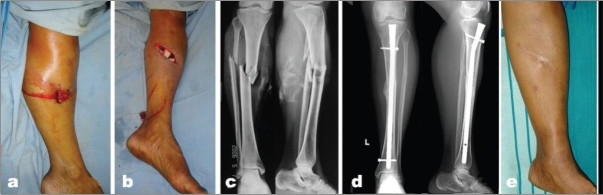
(a & b) Clinical photograph showing open injury of the tibia with exposed bone. (c) X-ray anteroposterior and lateral views of leg bones showing comminuted fracture of tibia. As per the Ganga Hospital Score, the total score is less than 5 and the skin score is less than 3. (d) X-ray anteroposterior and lateral view of leg bones showing skeletal fixation and union. (e) Clinical photograph showing result after immediate skin closure and a thorough debridement leading to good result
